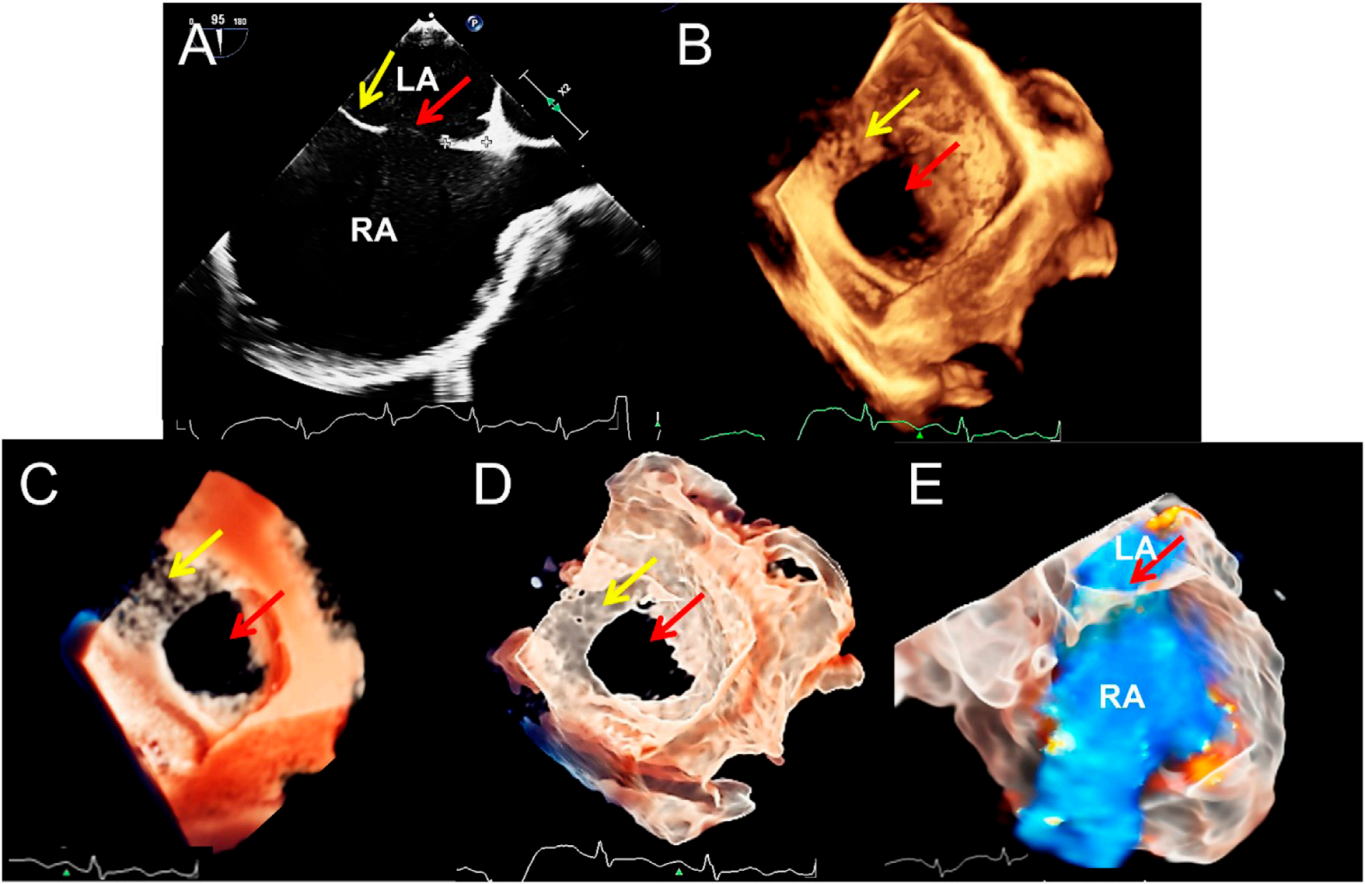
Transesophageal echocardiography of double atrial-superior and inferior vena cava section to diagnose atrial septal defect. (A–D). The 2D, traditional 3D, TrueVue Light, and TrueVue Glass show the secondary type of atrial septal defect (25 mm × 23 mm, red arrows) and the upper soft, thin rim (yellow arrows), respectively. Figure (B–D) is a direct view of the atrial septum from the left atrium perspective. (E). TrueVue Glass shows the spatial path of the atrial shunt from left to right. 2D, two-dimensional; 3D, three-dimensional; LA, left atrium; RA, right atrium.
